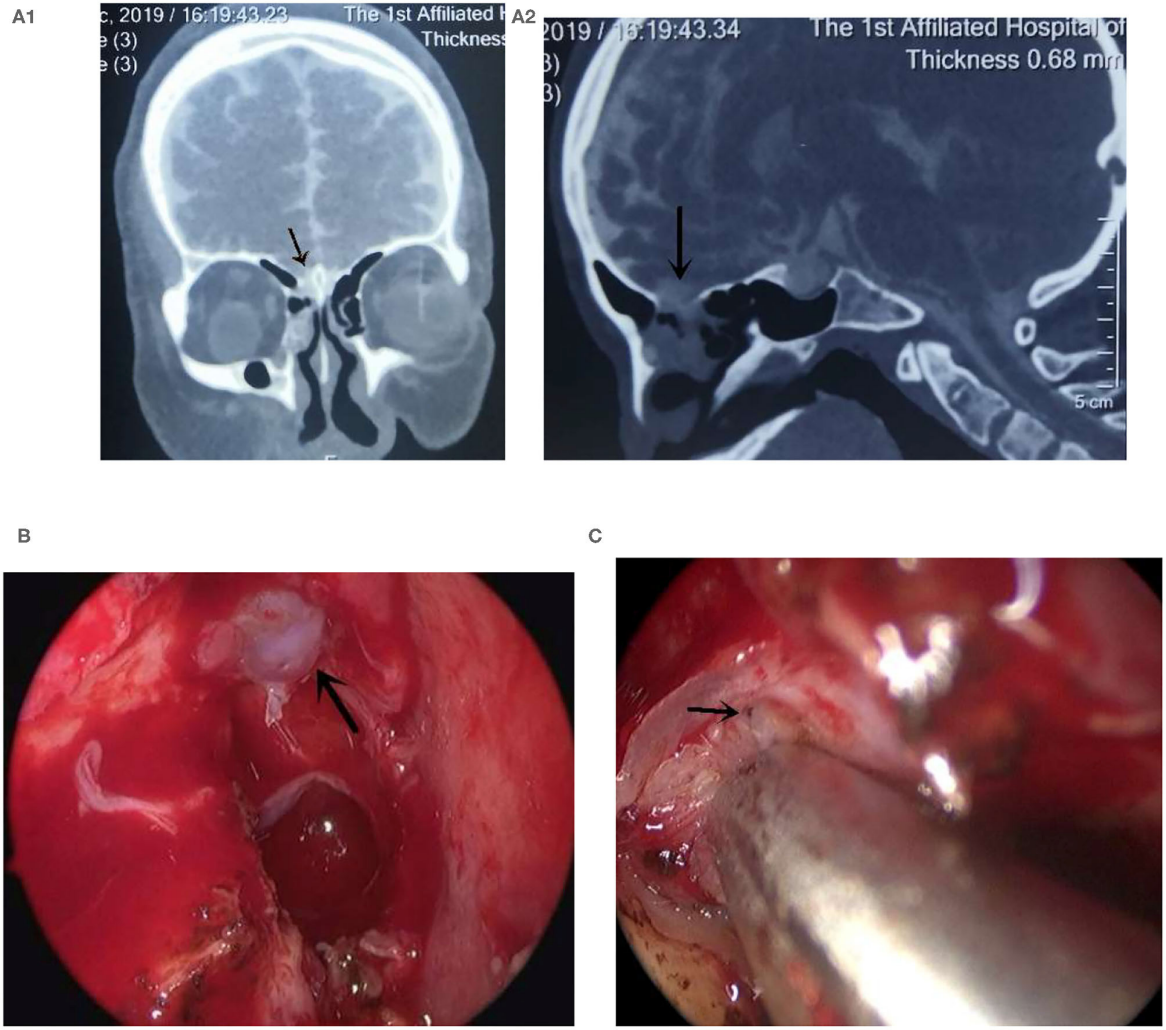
The high-flow leak was repaired endoscopically. (A) The CTC examination showed that the leak was located in the right ethmoid pate, (A1) the coronal CT, (A2) the sagittal CT, arrow showed the leak; (B) Arrow showed the edematous mucosa; (C) Exposed “pinpoint” leak after the injection of saline through lumbar drainage indicated by the arrow.
